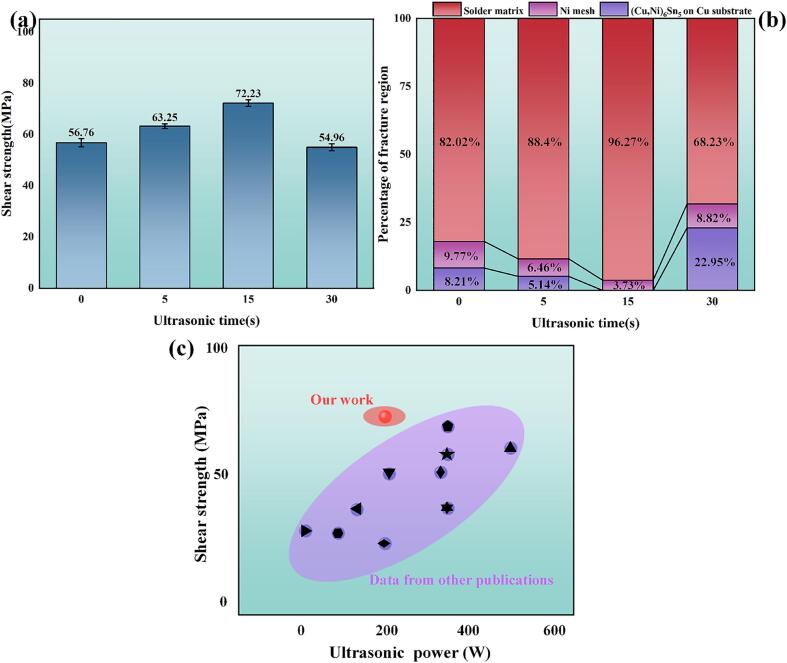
(a) Joint shear strength at various ultrasonic treatment durations, (b) proportional distribution of fracture area types, and (c) comparison between the current experimental results and those reported in the literature: [23], [24], [25], [26], [27], [11], [28], [22], [29], [30].
